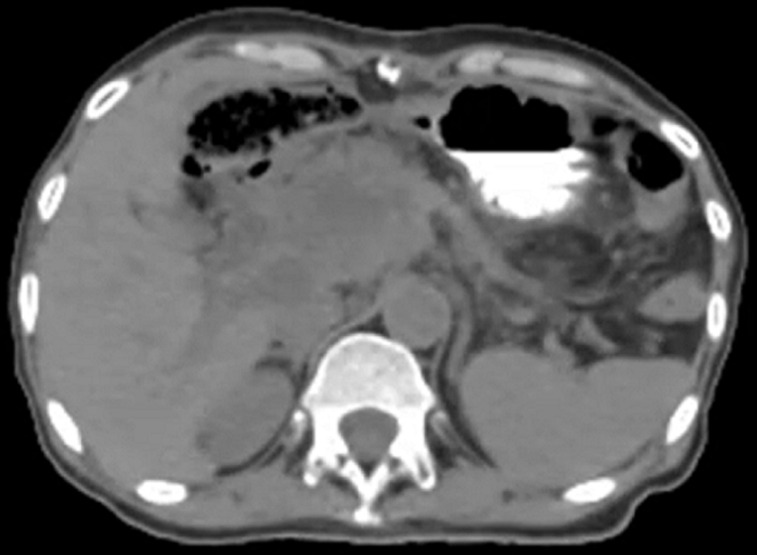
abdominal CT scan showing a hypodense pancreatic mass
